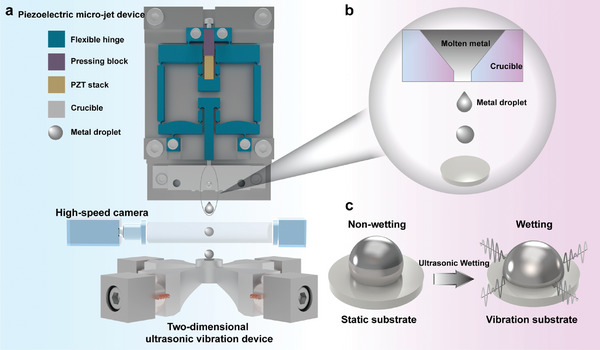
The experimental setup for the generation and deposition of metal droplets. a) Experimental setup based on the piezoelectric micro‐jet device and the 2D ultrasonic vibration deposition device. b) Generation of the metal droplet. c) The wettability of the metal droplet on the static substrate and vibration substrate.
